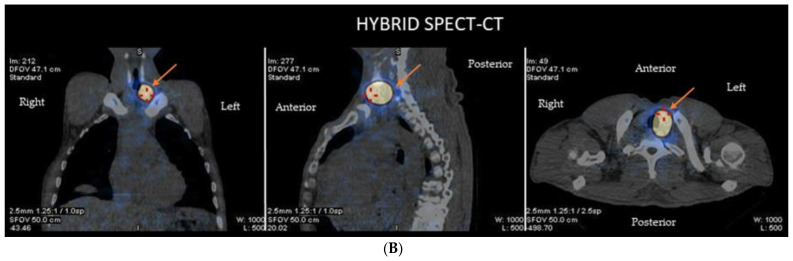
(A) A whole-body scan and planar images of a 42-year-old male with thyroid cancer who had initial radioactive iodine (RAI) therapy is depicted. The underlying principle of ^131^I thyroid therapy consists of the ablation of postoperative thyroid tissue residuals following thyroidectomy and treating metastatic disease after incomplete or total thyroidectomy. Dosing over 30 mCi results in obliteration of the thyroid by the beta particles causing ionization. This patient received 125 mCi ^131^I ablation therapy. Due to Hurtle cell carcinoma (oncocytic cell follicular thyroid carcinoma) in left lobe and 8 days post-therapy, whole-body imaging was performed to look for any nodal/distant metastases. A region of intense uptake was observed in the lower cervical region, on the left of the midline, giving an image similar to a star known as a “star sign” that is seen in the anterior images (this is due to the high uptake of RAI of the remnant thyroid tissue after surgery—arrow). The high uptake of ^131^I in the remnant thyroid tissue is also seen in the posterior images (arrowheads). No abnormal accumulation was noted elsewhere. (B) This figure demonstrates the hybrid single-photon emission tomography (SPECT) and low-dose computed tomography (CT) images for anatomical verification of the finding and disclosure of any nodal uptake near the star sign. The combination of CT with the iodine scan accurately localizes the thyroid tissue uptake (arrow) from the metastatic lesions, providing a three-dimensional view.
